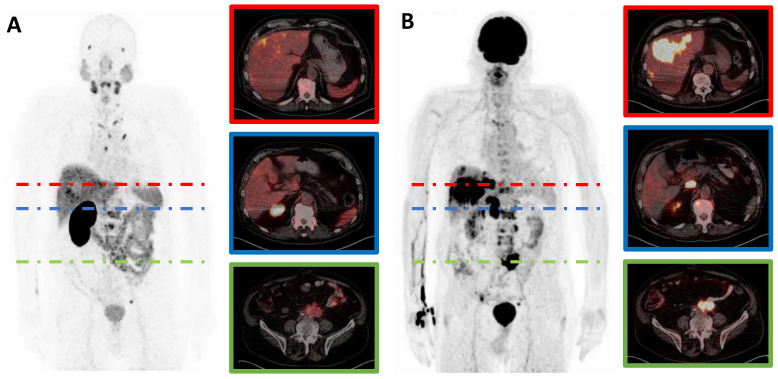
[^68^Ga]Ga-PSMA-11 (A) and [^18^F]FDG (B) PET/CT images of a representative mCRPC patient with hepatic (red) and lymphonodular (blue and green) mismatch lesions. Note: mCRPC, metastatic castration-resistant prostate cancer.
